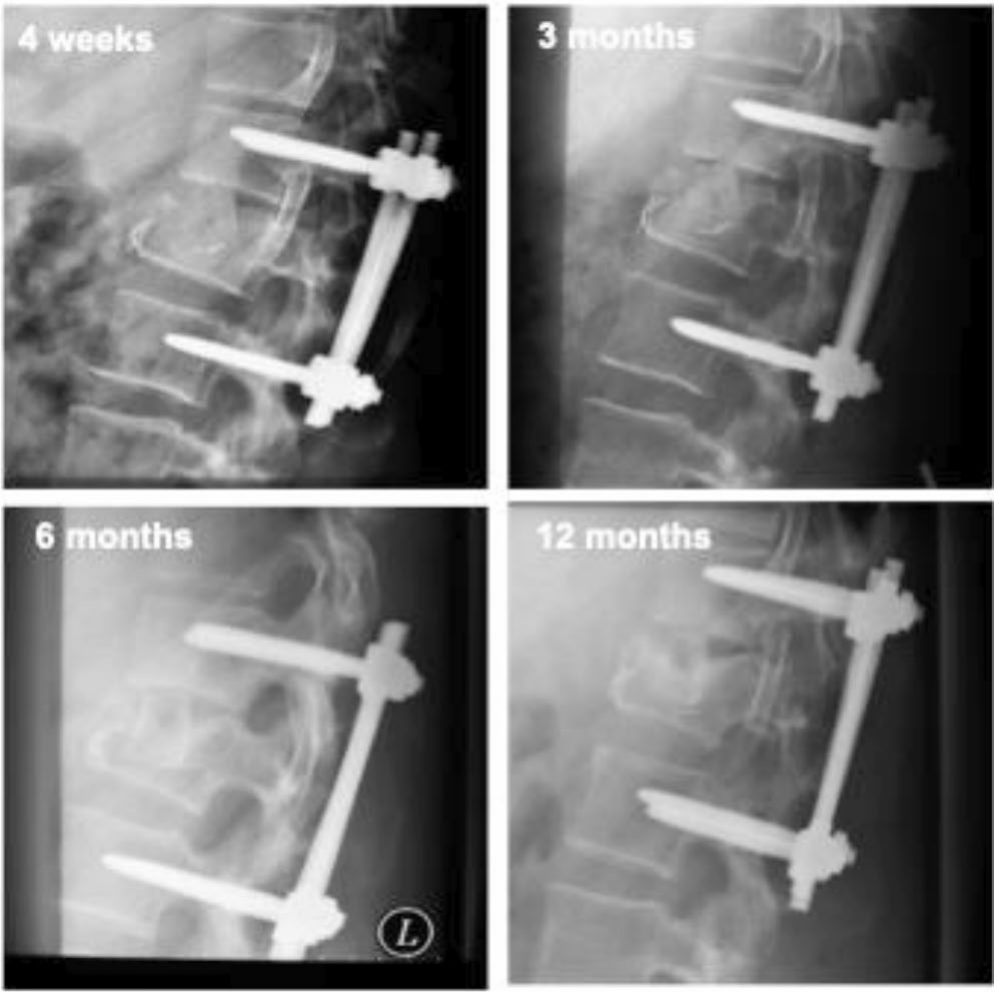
Plain radiographs documenting ongoing necrosis of bone graft and lack of fusion. Fracture Type AO A 3. Male, 41y 4 weeks, 3 months, 6 months, 12 months
